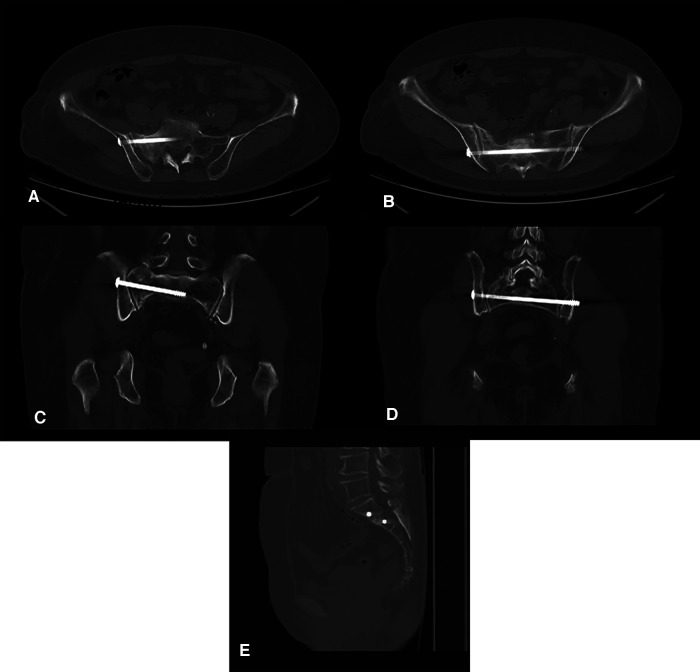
Imaging verification with rail-bound CT during operation. (A,B) Horizontal view. (C,D) Coronal view. (E) Sagittal view.
